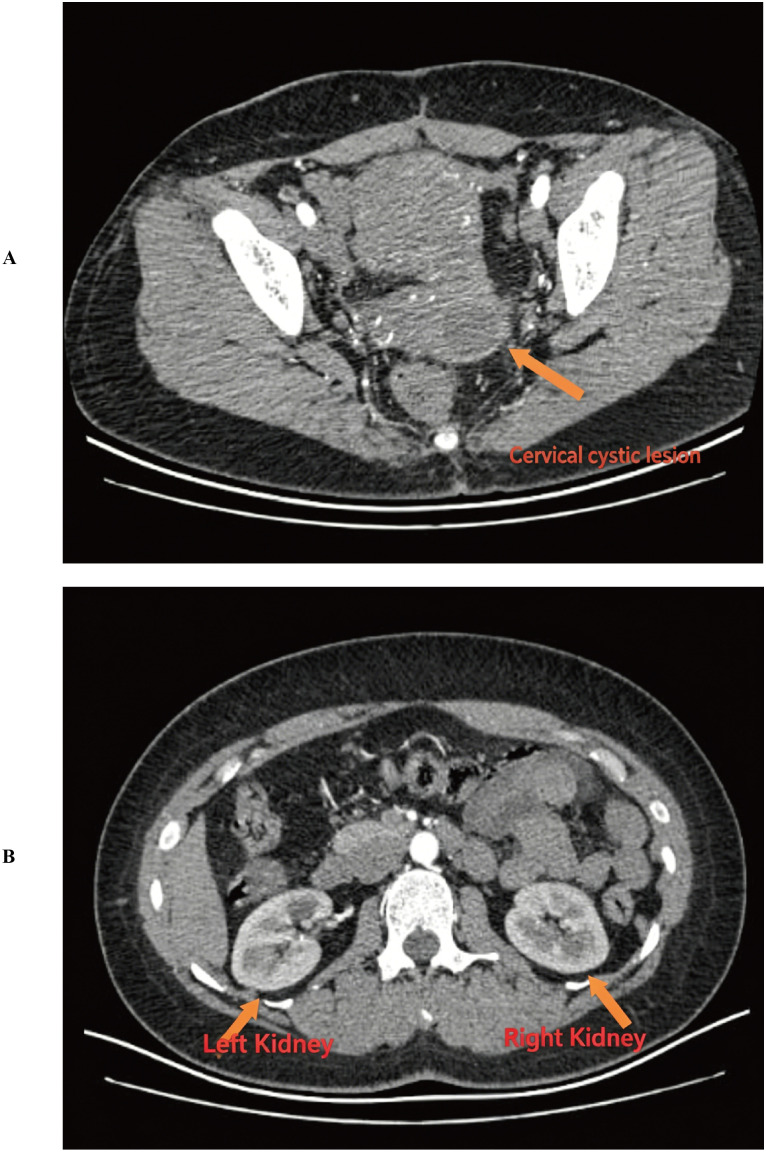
Postoperative contrast-enhanced computed tomography (CT) of the lower abdomen performed 2.5 months after myomectomy. (A) Axial image shows a well-defined, non-enhancing cystic lesion (arrowhead) at the left lateral aspect of the cervix, compatible with a benign nabothian cyst. (B) Coronal image demonstrates normal morphology of both kidneys (arrows) with no evidence of renal masses or other abnormalities.
